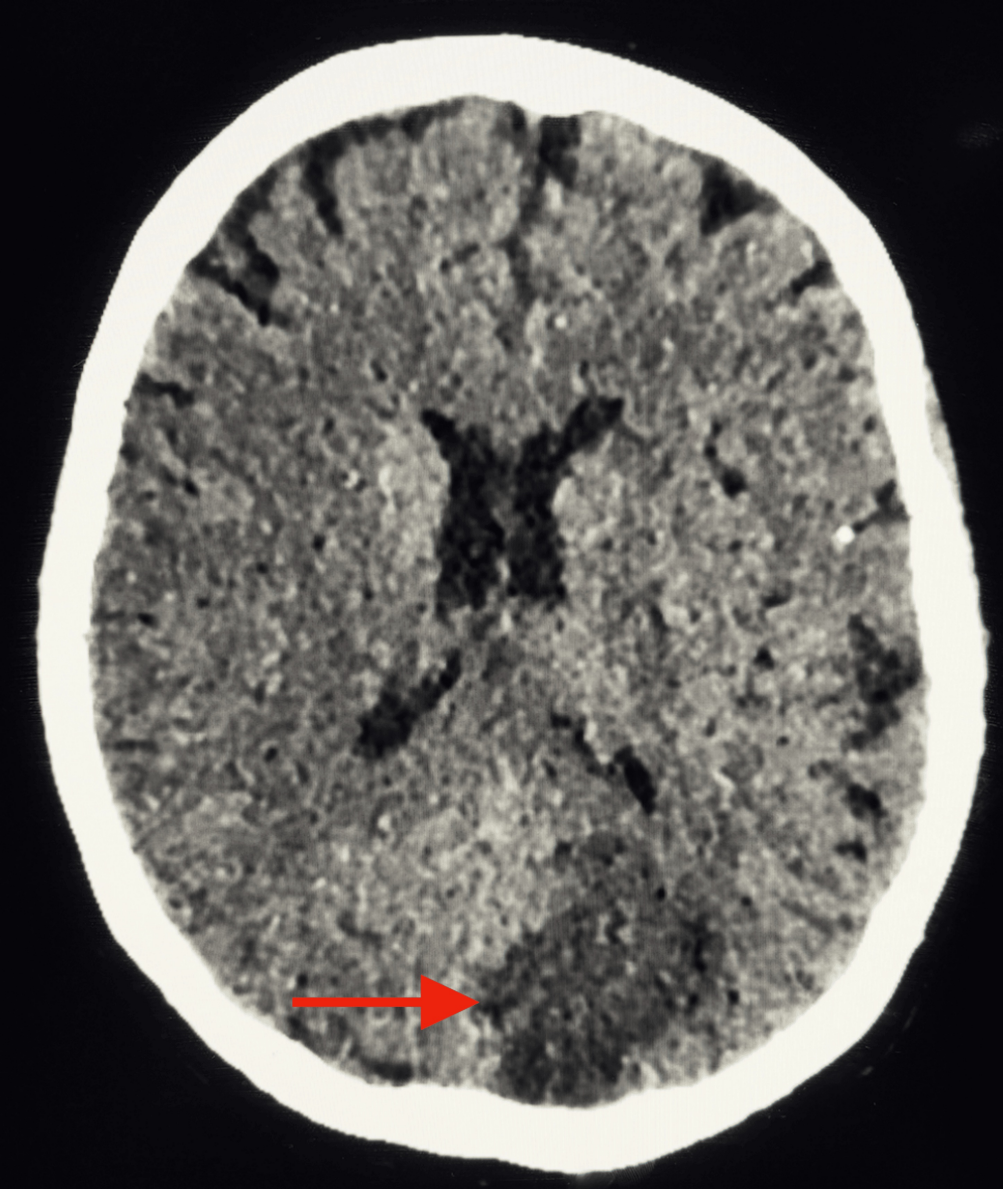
Transverse section of a brain CT scan demonstrating postoperative ischemic lesions (red arrow).
